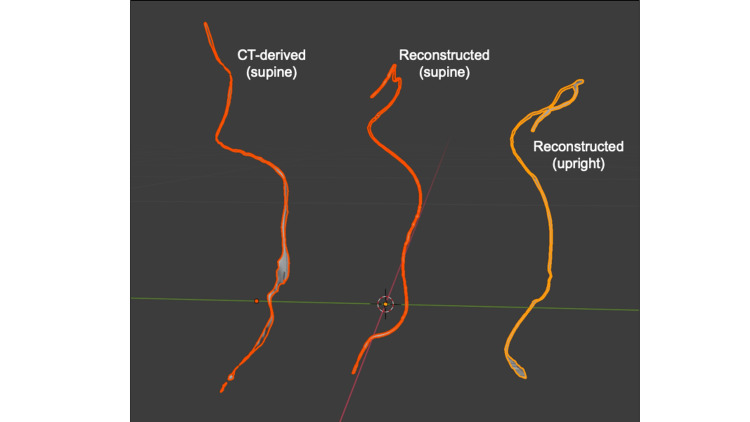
Visual comparison of the reconstructed lead trajectories. CT-derived and two reconstructed lead trajectories were aligned in the Unity workspace. The green line indicates the frontal axis, and the red line indicates the lateral axis.
